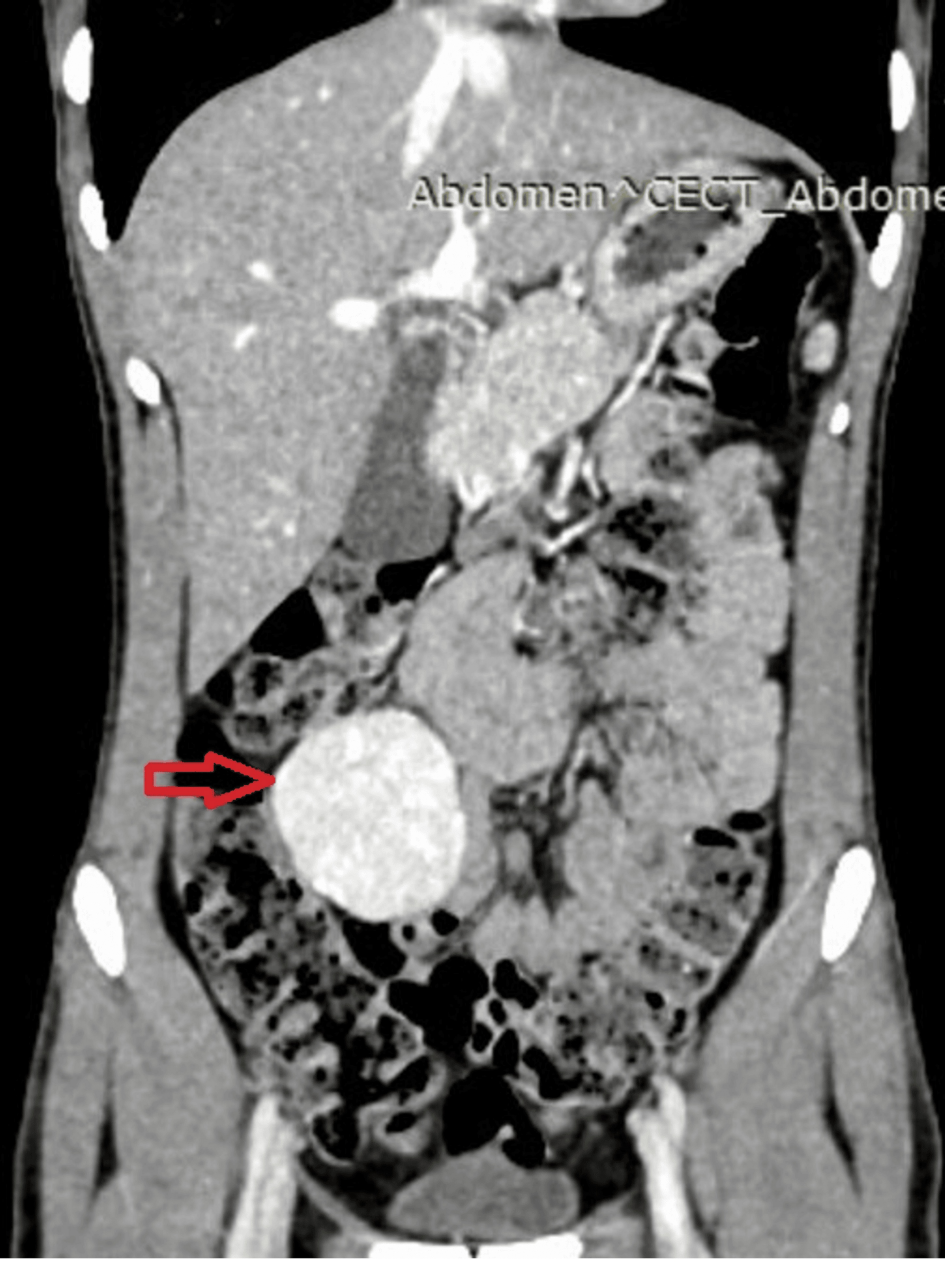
Coronal CECT abdomen showing intensely enhancing mesenteric mass in right iliac fossa (4.3×3.2 cm, red arrow) abutting small bowel loops. CECT: contrast-enhanced computed tomography
